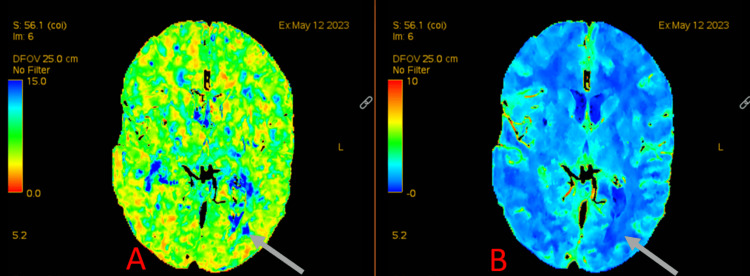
CT angiography perfusion showing mean transit time (A) and cerebral blood volume (B) A. Demonstrates an area of increased mean transit time in the left parieto-occipital region. B. Demonstrates no increased signal on cerebral blood volume imaging in the same area. This indicates an area of ischemic penumbra in this region CT: computed tomography
